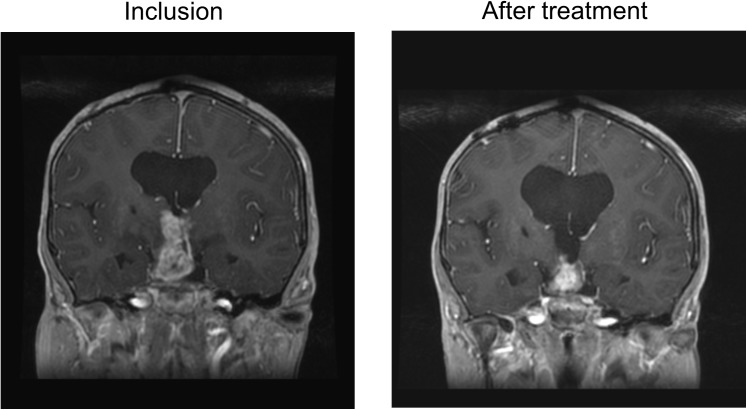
MRI of a patient with LGG at inclusion and after 4 months of MC.
